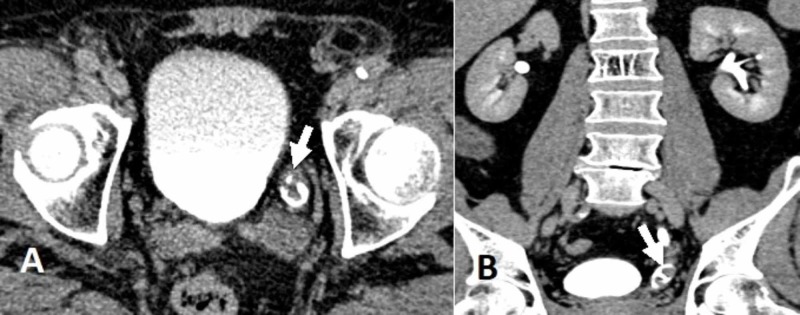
Enhanced computed tomography scan during the excretory phase (CT urography) (A) filling defect noted within the distal left ureter (arrow) on the axial view; (B) Coronal view showing a filling defect within the distal left ureter (arrow), suggestive of urothelial carcinoma
